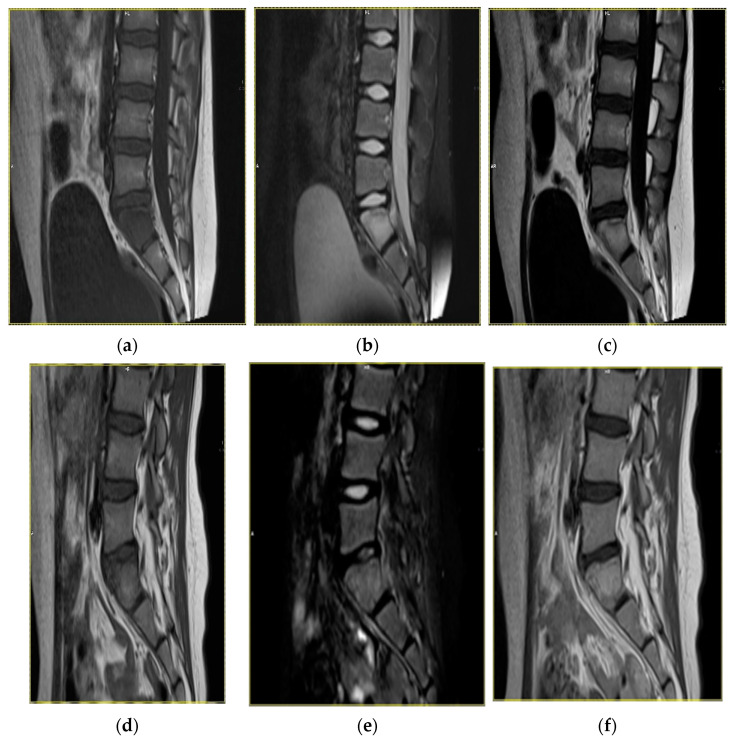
Magnetic resonance images in a 9-year-old female patient with inflammatory lesions within S1 sacral vertebra. Status before treatment: T1-weighted sequence (a), T2-weighted sequence (b), T1-weighted sequence with contrast enhancement (c). Status after an 8-month follow-up, with regression of the inflammatory lesions: T1-weighted sequence (d), T2-weighted sequence (e), T1-weighted sequence with contrast enhancement (f).
